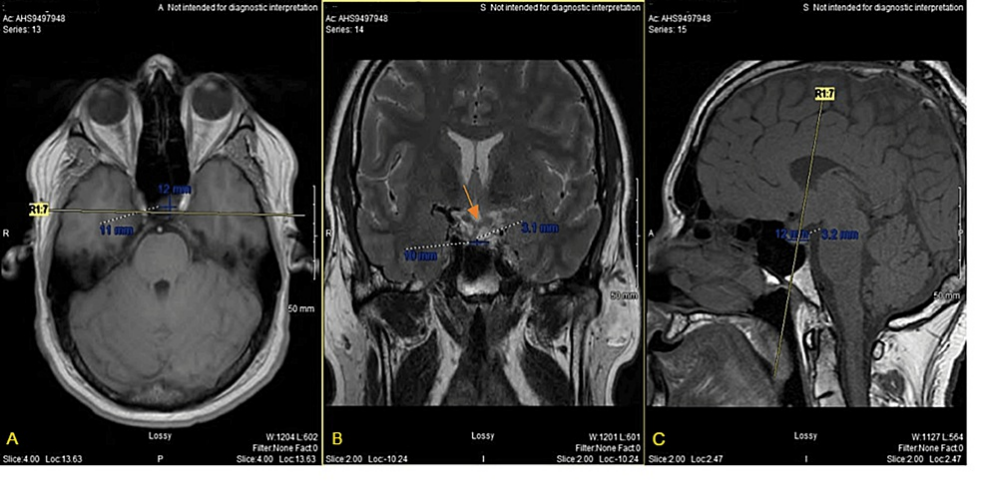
Follow-up MRI displaying near complete regression of sellar mass. (A) Axial T1, (B) coronal T2, and (C) sagittal T1 views of tumor measuring 12 x 10 x 3.1 mm (L x W x H) in the most recent MRI. This displays regression of the tumor with complete visualization of the optic chiasm (arrow in B).
